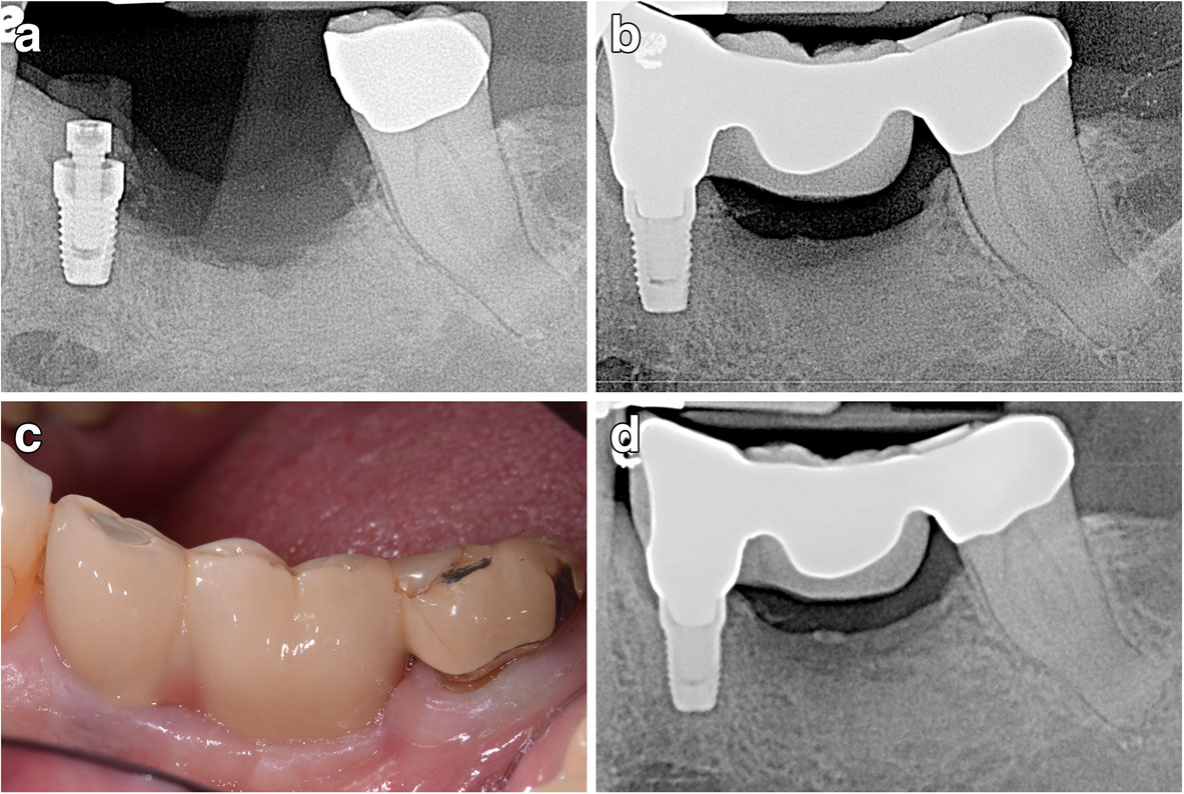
a Radiographic image of a 3.5-mm-wide by 7-mm-length dental implant at the time of its placement at the mandibular left second premolar in a 63-year-old Caucasian female. b Radiographic image of a three-unit fixed partial denture upon its initial placement. The dental implant is the anterior abutment with the prosthesis screw retained to it. The distal abutment is the mandibular left second molar. A coping has been placed in the event of intrusion. c Clinical image of the three-unit fixed partial denture at 58 months post-loading. Soft tissue health has been maintained around both the tooth and the dental implant. d Radiograph exposed at 58 months demonstrating the steady state of bone on both the dental implant and the natural tooth abutments. There is no suggestion of any intrusion occurring with the mandibular left second molar
